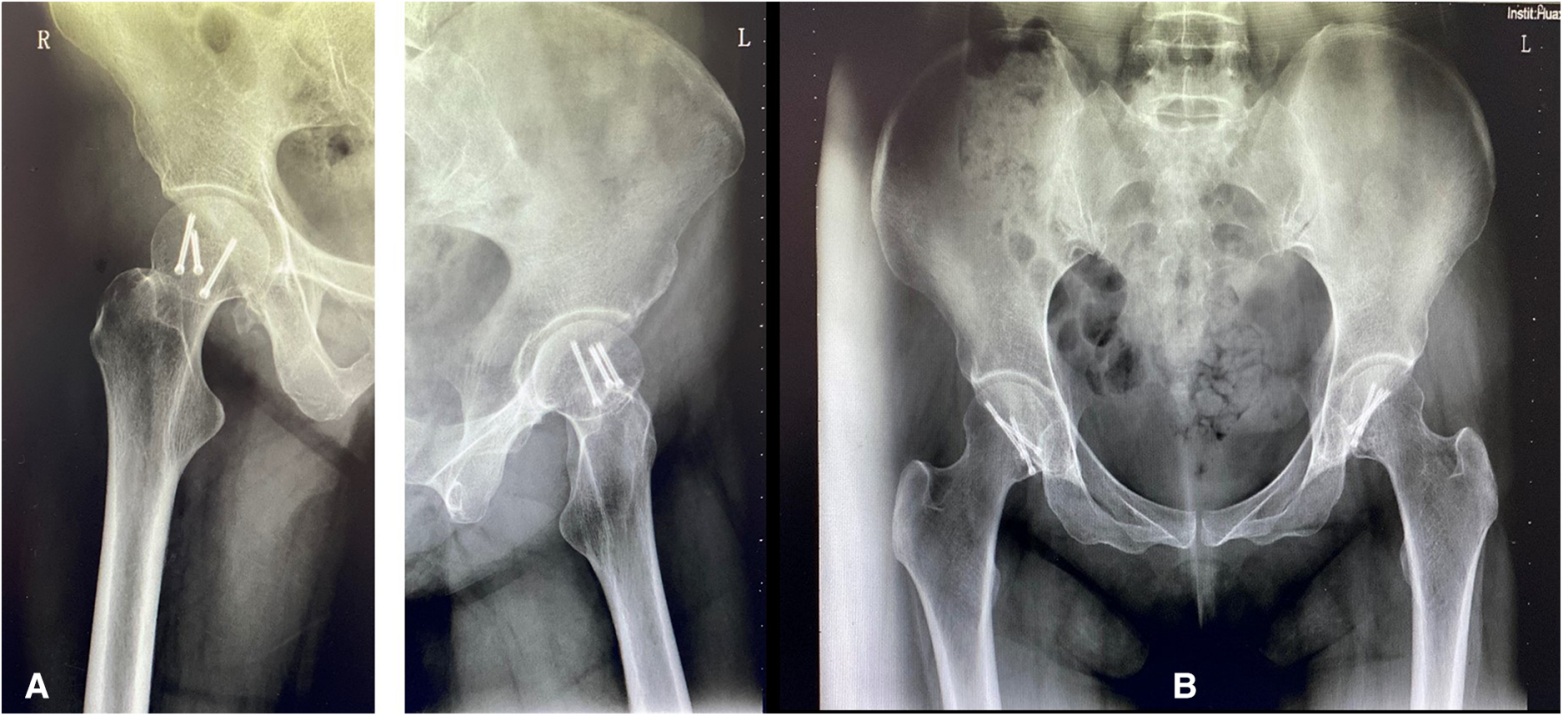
(A) X-ray, right and left lateral view (B) Plain x-ray AP view of a pelvis showing bilateral internal fixation of femoral heads during the 5-year follow-up without sign of osteonecrosis and post-traumatic osteoarthritis and heterotopic ossification.
